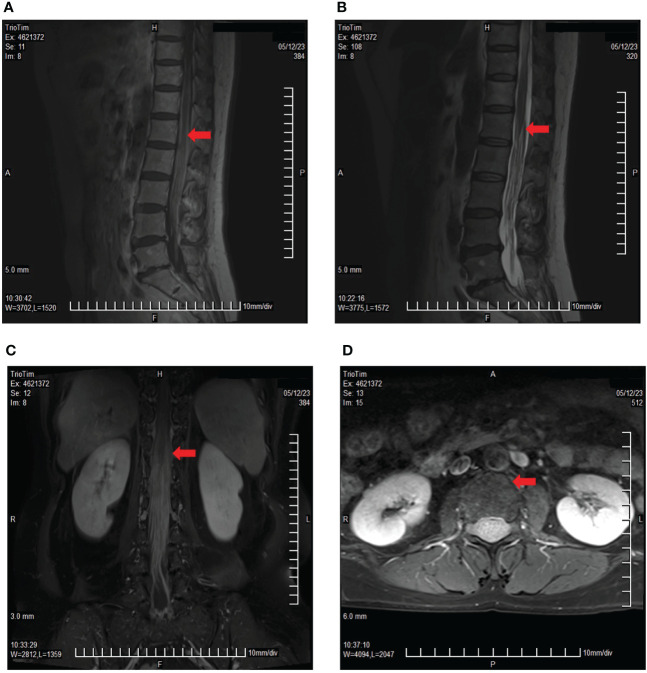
Lumbar spine MRI showing multiple cord shadows at the cauda equina, which were significantly thickened and increased, indicating the rapid progression of the disease. (A) Sagittal T1 sequence; (B) Sagittal T2 sequence; (C) Coronal enhancement sequence; (D) Transverse enhancement sequence (arrows indicate tumor foci).
